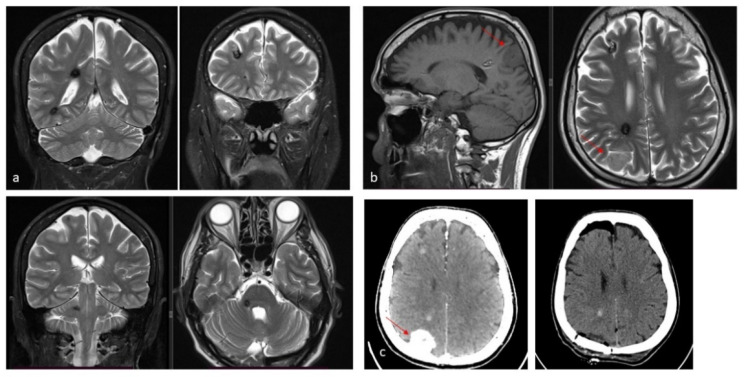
(a). Axial and coronal T2-weighted MRI of a 54-year-old patient affected by an incidentally discovered familial form of CM. Multiple asymptomatic CMs were observed supratentorially, infratentorially, and at the brainstem. (b). Sagittal T1-weighted and axial T2-weighted MRI showing a concomitant symptomatic parieto-occipital meningioma, which required surgery (red arrow). (c). Pre- and postoperative CT scan with contrast showing total removal of the meningioma (red arrow) and multiple hyperdense lesions corresponding to CMs.
